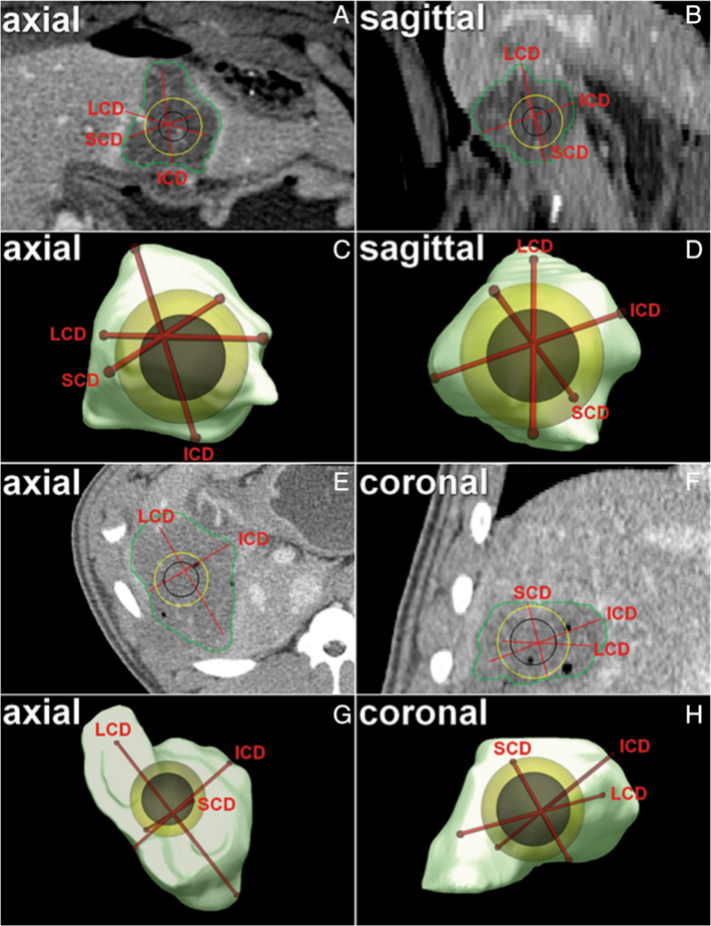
Technique A (Semi-automated Software Prototype for CT 3d Rendering with the “Chebyshev Center Concept” implemented) - Image Example. A-D Axial image plane (A) and sagittal image plane (B) as well as corresponding volume rendering (C, D) – IRE Protocol 2. E-H Axial image plane (E) and coronal image plane (F) as well as corresponding volume rendering (G, H) – IRE Protocol 3. Note: after manual segmentation of the treatment zone (green), long, intermediate and short diameter (LCD, ICD and SCD) as well as the largest inscribed sphere within the treatment zone (yellow) and the largest possible treatable tumor sphere (black) were defined automatically (in Figure 4E, SCD is not indicated since its craniocaudal course). The barycenter offset is the distance between the barycenter of the treatment zone (intersection point of long, intermediate and short diameter) and the “Chebyshev Center” (center of the largest inscribed sphere within the treatment zone = center of the largest possible treatable tumor sphere). Observe the conspicuous eccentricity of the “Chebyshev Center” within the treatment zone (which is quantified by the barycenter offset) on standard image planes (B and E) which is not assessable with standard CT 3d analysis (Figure 5). Visualization and quantification of size and position of the largest inscribed sphere within the treatment zone (as well as of the largest possible treatable tumor sphere) can be relevant for acute treatment control after IRE (e.g. confirmation of the intended safety margin size).
